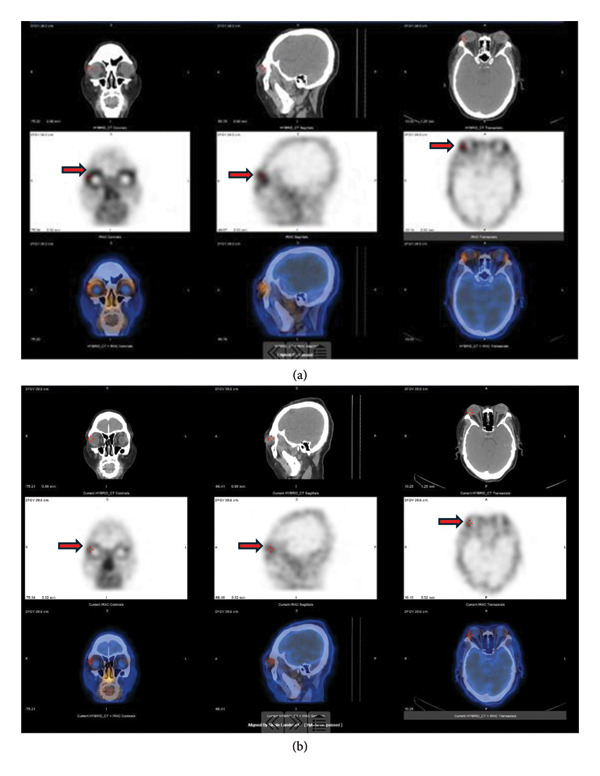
Pre‐ and post‐treatment nuclear medicine orbital imaging. Pre‐ and post‐treatment nuclear medicine orbital imaging (99mTc‐DTPA SPECT/CT). (a) Pretreatment images showing moderate to high uptake in orbital tissues indicating inflammation (arrows indicate areas of increased tracer uptake). (b) Post‐treatment images at 16 weeks showing reduced uptake, consistent with decreased inflammatory activity. Five patients (62.5%) showed improvement from moderate to mild inflammatory activity.
